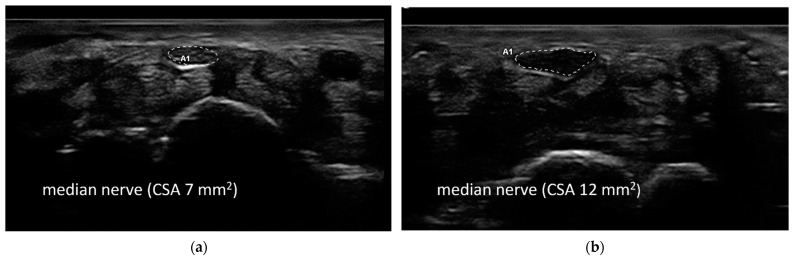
Axial ultrasonographic images of the median nerve at the wrist in a healthy control (a) (cross-sectional area 7 mm^2^) and in a patient with electrodiagnostically evaluated carpal tunnel syndrome (b) (cross-sectional area 12 mm^2^).
